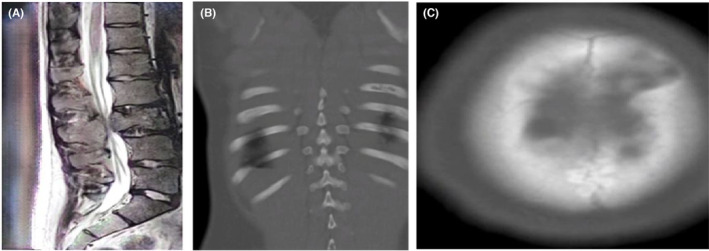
(A) MRI showing a destructive lesion at L3 vertebra. (B) CT‐scan chest showing osteolytic lesion in the left third Rib. (C) CT‐scan head showing osteolytic lesion in left parasagittal calvarium.
